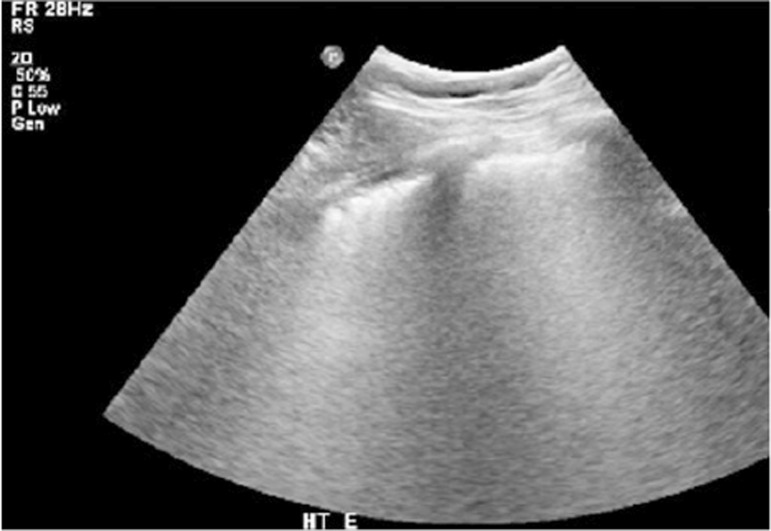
Ultrasonographic ground-glass: multiple confluent B-lines corresponding to the “ground glass” pattern on computed tomography
